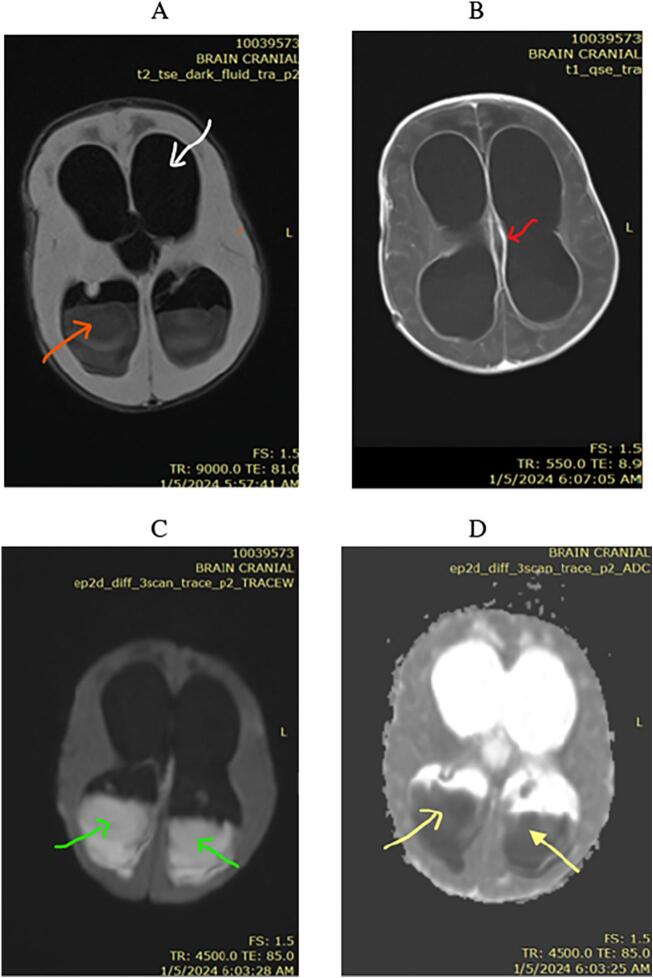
a. FLAIR ventriculomegaly (white arrow) with hyperintense intraventricular material (red arrow). b. Post-contrast T1-weighted sequences reveal Enhancement of the ventricular walls (red arrow). c. DWI- demonstrates marked hyperintensity (green arrows). d. ADC; Low apparent diffusion coefficient (yellow arrows).
